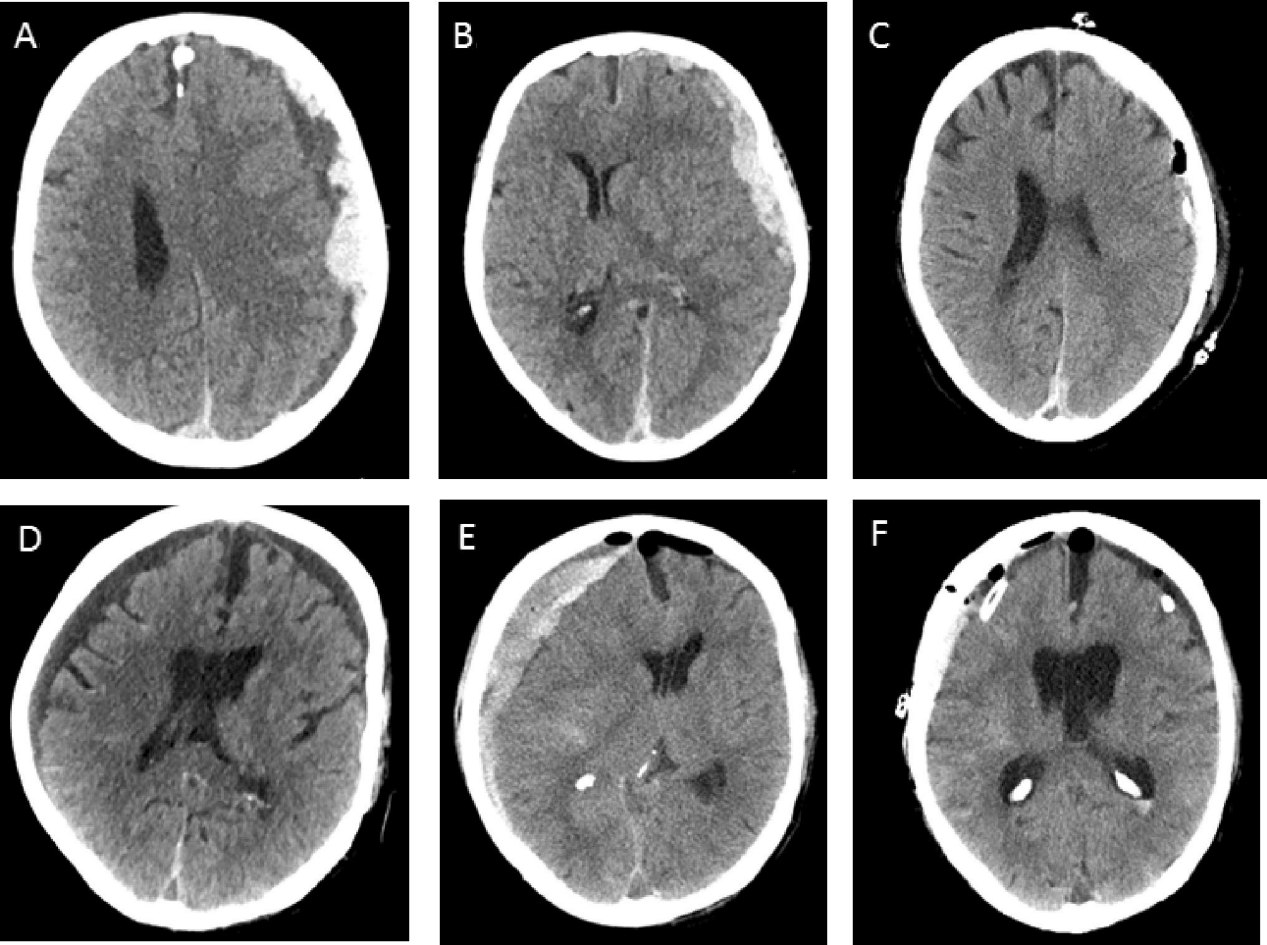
a, b Axial slice of a native CT-scan, showing acute bleeding into the previously detected cSDH, which was initially treated conservatively, due to anticoagulant intake and minimal clinical manifestation. c Postoperative axial slice of a native CT-scan, showing good postoperative SDH relief after burr hole evacuation. d Axial slice of a native CT-scan, showing a bilateral cSDH before bilateral burr hole evacuation. e Axial slice of a native CT-scan showing acute bleeding on the right side, postoperatively. f Axial slice of a native CT scan, after resurgery via craniotomy, on the right side. CT, computer tomography; cSDH, chronic subdural hematoma
